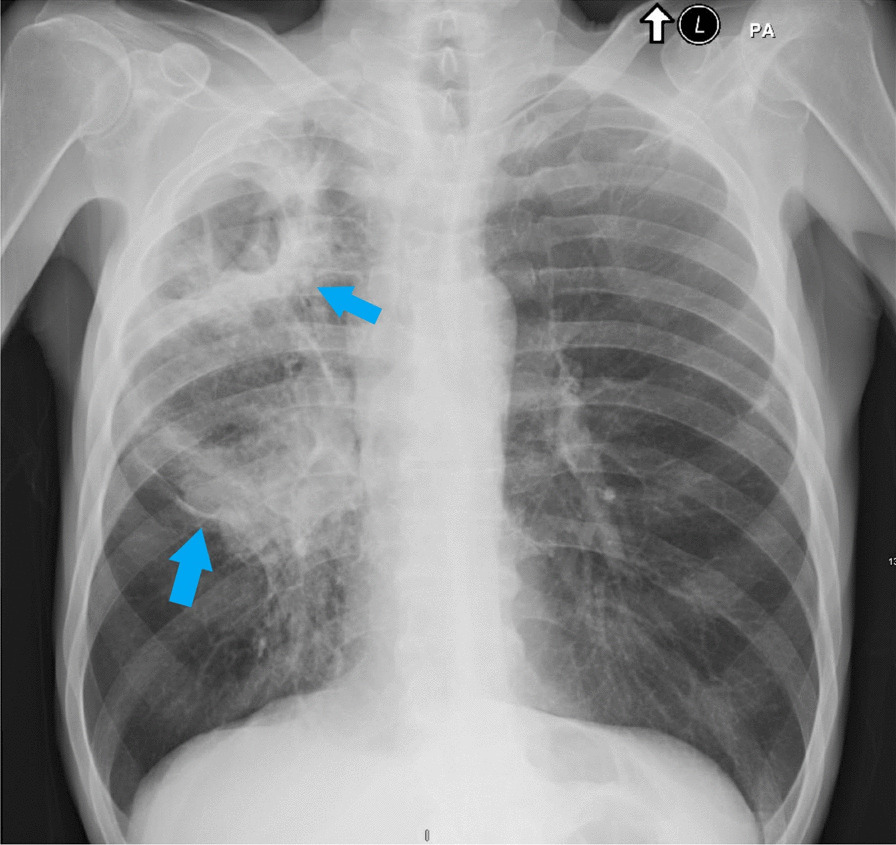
Chest radiograph showing large cavitary lesion in the right upper lung with right hilar mass (blue arrows)
